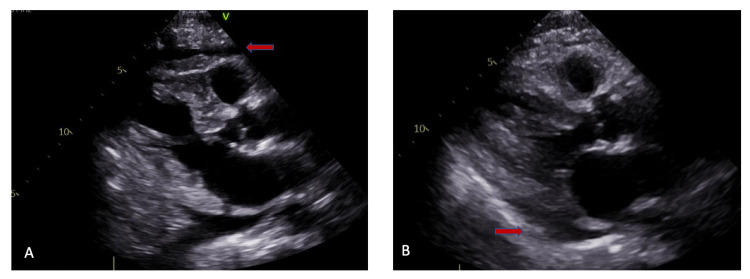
Two-dimensional (2D) echocardiography - (A) day 1 and (B) day 3 Day 1 - there is moderate circumferential pericardial effusion; day 3 - there is trace pericardial effusion.
